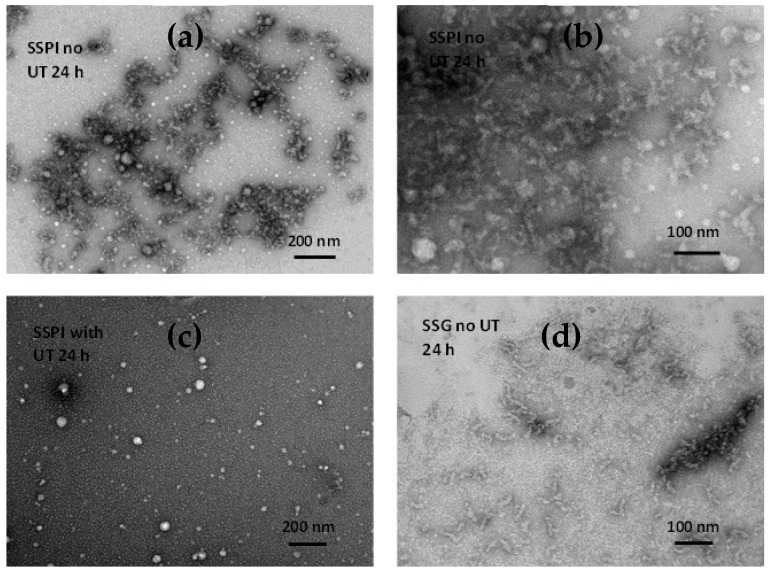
TEM images of sesame protein amyloid species after fibrillation for 24 h at pH 2 and 80 °C: (a) SSPI amyloid species prepared from 3% w/w protein solution without ultrasonication treatment; (b) Image of SSPI amyloid species at higher magnification; (c) SSPI amyloid species prepared from 3% *w*/*w* protein solution with ultrasonication treatment; (d) SSG amyloid species prepared from 3% *w*/*w* protein solution without ultrasonication treatment.
